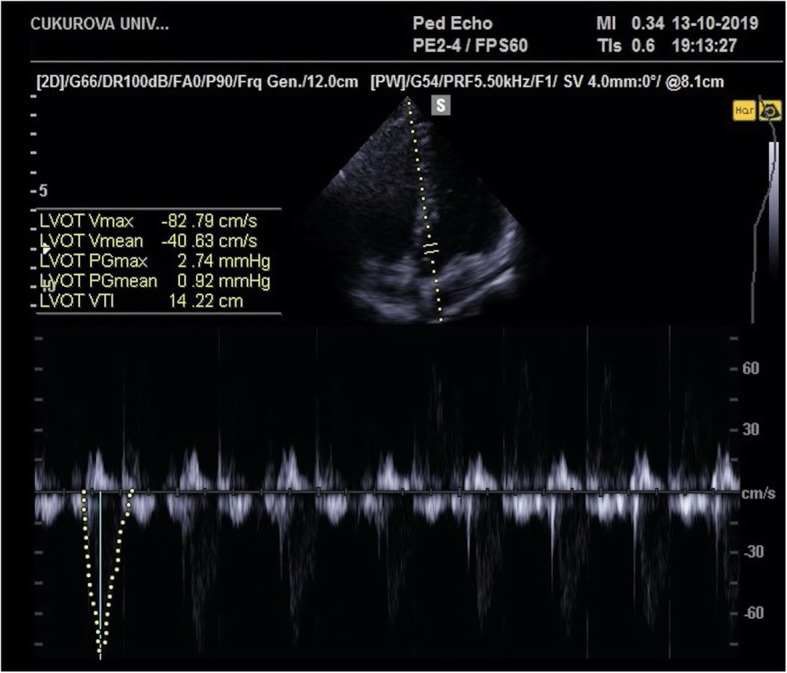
LVOT-VTI measurement in the apical five chamber image
